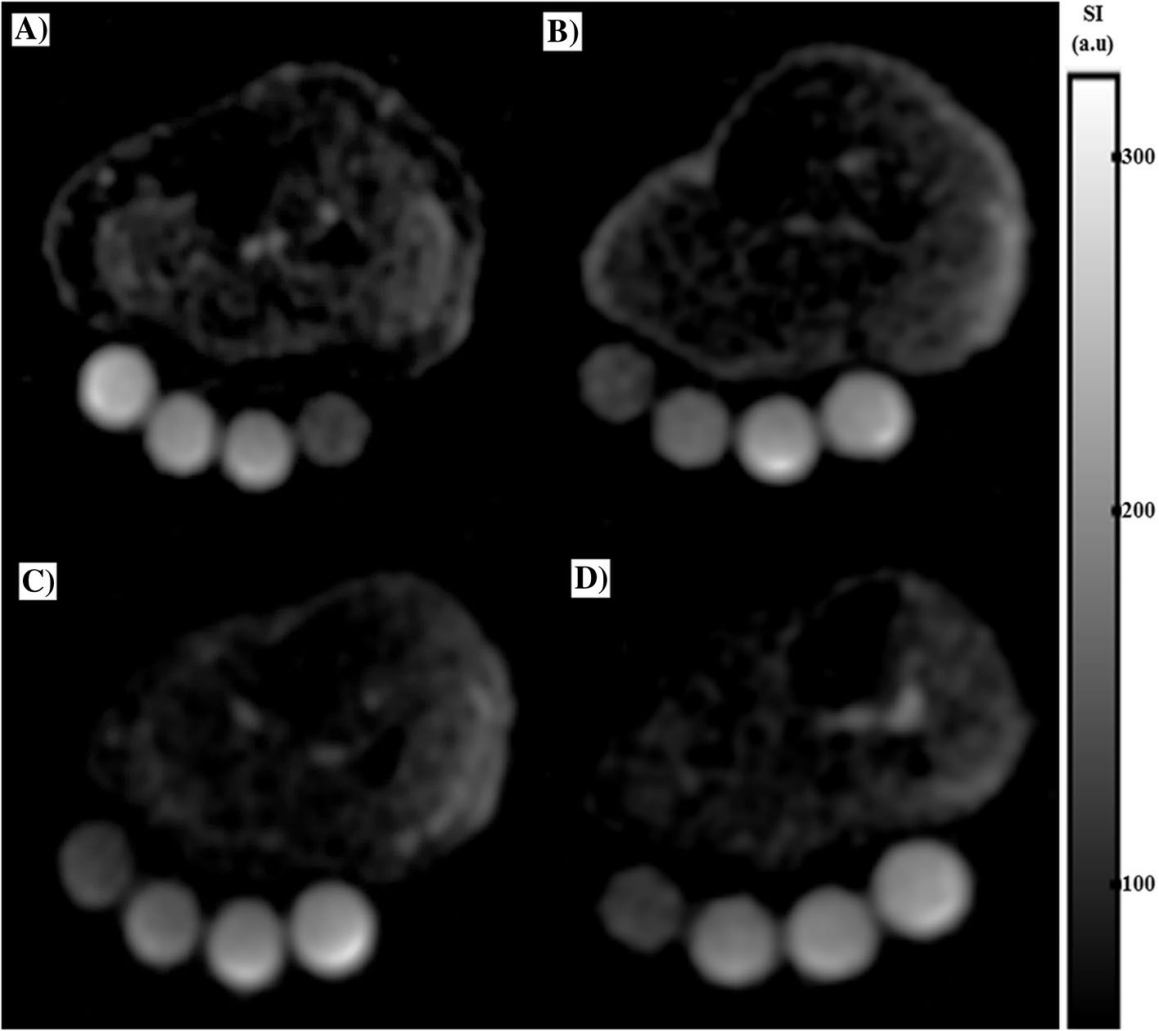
Sodium magnetic resonance imaging (^23^Na-MRI) of lower calf of the right leg. ^23^Na-MRI in the transversal orientation of (A) a 41-year-old healthy woman, and (B) in a 32-year-old healthy man. ^23^Na-MRI in the axial orientation of (C) a 49-year-old woman (D) and a 33-year-old man with a diagnosis of Addison’s disease; gray scale bar shows image signal intensity (SI) in arbitrary units (a.u.)
